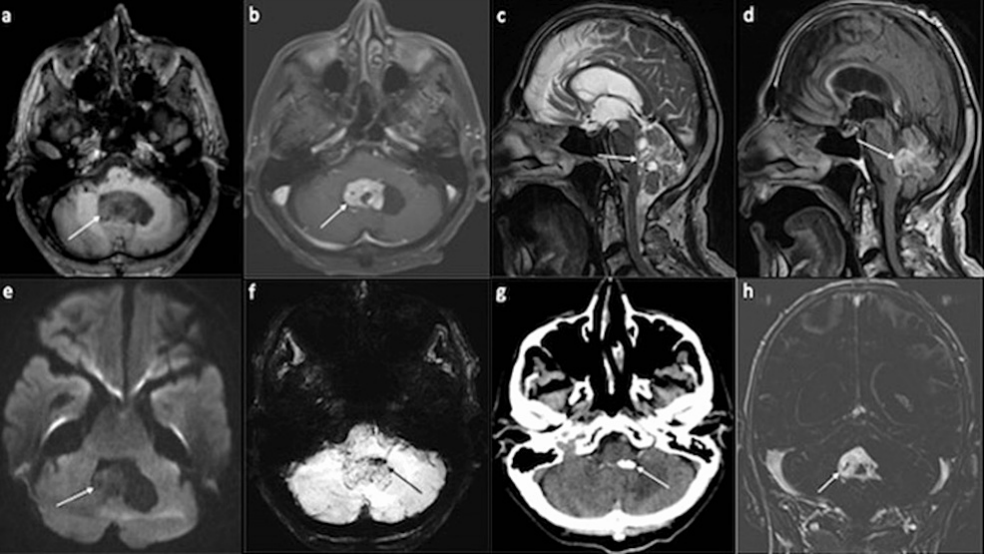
Preoperative magnetic resonance imaging and computed tomography Preoperative T1-weighted±contrast medium MRI revealed a T1-hypointense (Figure 1a) tumor with contrast-enhancement (Figure 1b) located in the fourth ventricle. On T2-weighted (Figure 1c) and fluid-attenuated inversion recovery (FLAIR) (Figure 1d) sequences, the tumor appeared hyperintense. A signal drop in apparent diffusion coefficient (ADC) mapping without restriction in diffusion-weighted imaging (DWI) was also noted (Figure 1e). Susceptibility-weighted imaging (SWI) was indicative of intratumoral calcifications (Figure 1f), which were confirmed by cCT (Figure 1g). T1-weighted MRI with fat-subtracted sequences showed no presence of significant intratumoral fat contents (Figure 1h).
